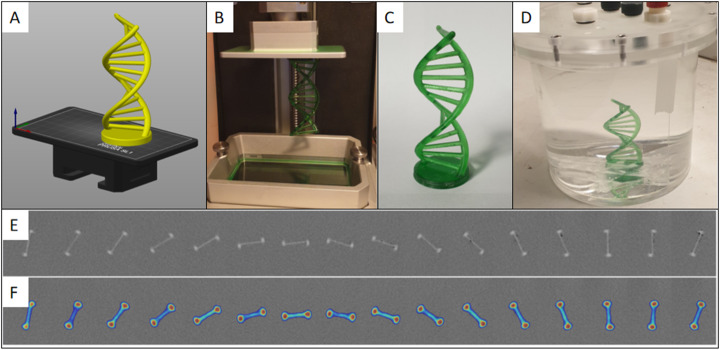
Helix model prepared for printing in PrusaSlicer (a). Helix after printing (b). Helix after removal from build plate, washed, dried and cured (c). Helix mounted in a cylinder of water for imaging (d). Axial CT slices (e). Axial PET/CT slices (f)
